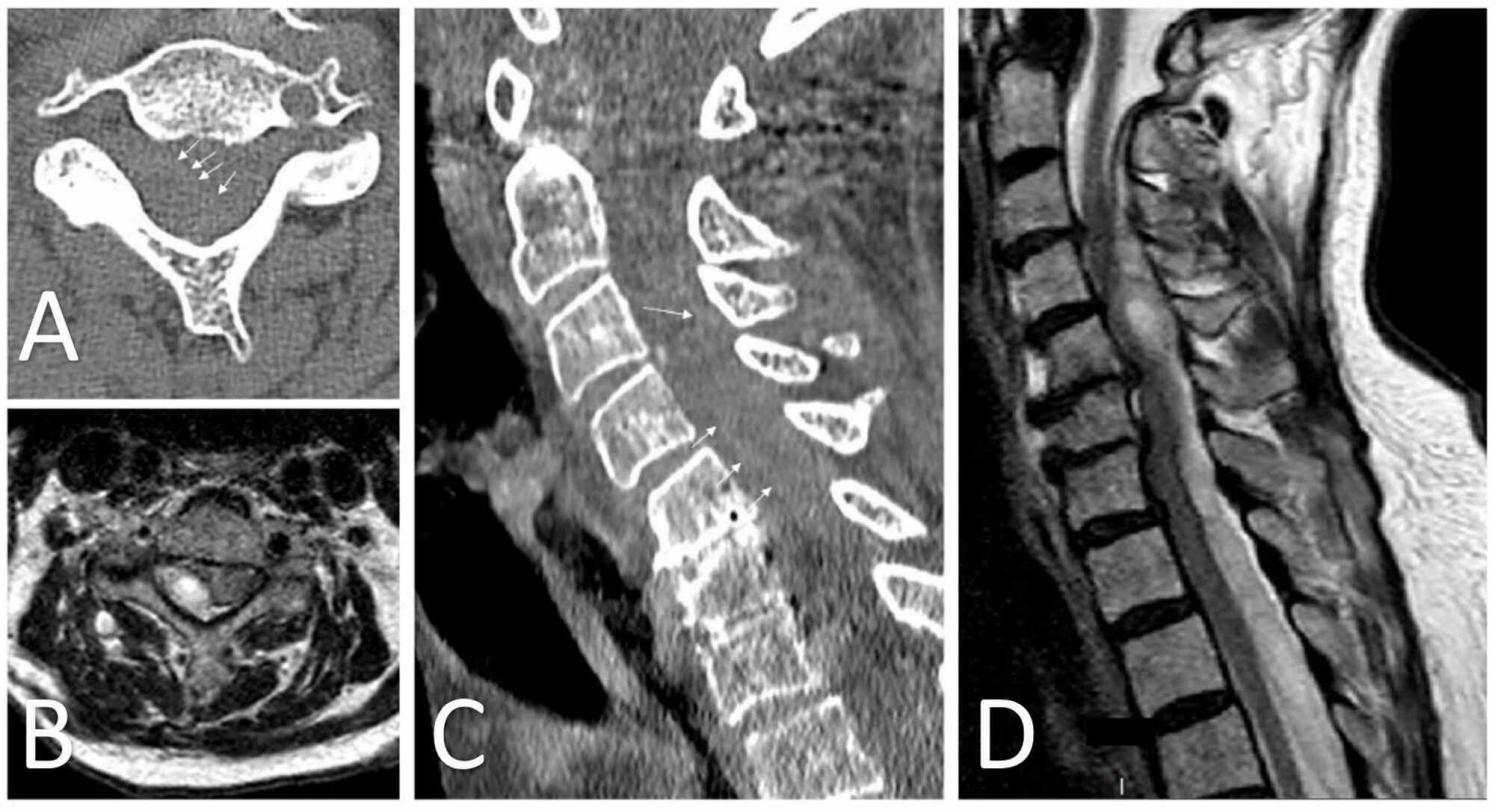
Acute epidural hematoma spanning from C2-T3 Axial (A) CT and (B) T2-weighted MRI demonstrating evidence of acute epidural fluid collection with central canal stenosis and spinal cord compression. Sagittal (C) CT and (D) T2-weighted MRI in the same patient. Hemorrhage is eccentric to the right, consistent with the patient’s more severe right-sided symptoms on presentation. White arrows demonstrate the hemorrhage border with the spinal cord on CT.
